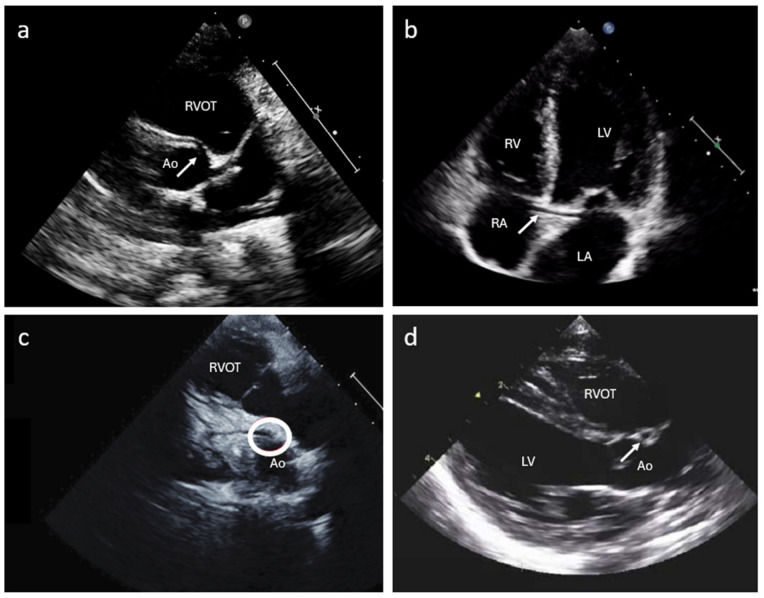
Ultrasound examination in coronary anomalies: (a) Right coronary artery from the left sinus of Valsalva in parasternal short-axis view (arrow). (b) Circumflex coronary artery from the right sinus of Valsalva with a retroaortic course in four-chamber view (arrow). (c) Single coronary artery from a modified parasternal short-axis view (circle). (d) The ring sign indicates a right coronary artery from the opposite sinus of Valsalva with a presumed intramural course on parasternal long-axis view (arrow). RVOT, right ventricular outflow tract; LV, left ventricle; RV, right ventricle; Ao, aorta, LA, left atrium; RA, right atrium.
